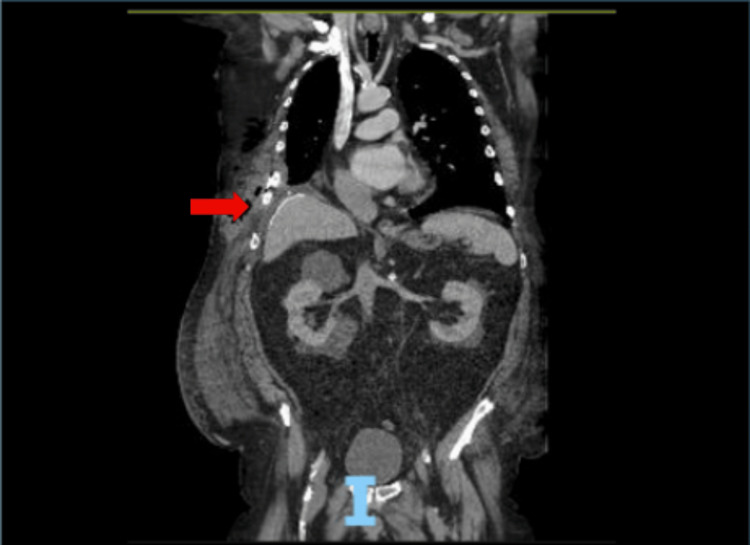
Coronal computed tomography image displaying diaphragmatic hernia repair with mesh, chest wall hernia repair, and approximation of the rib fractures Red arrow: Area of rib plating and diaphragmatic mesh repair.
